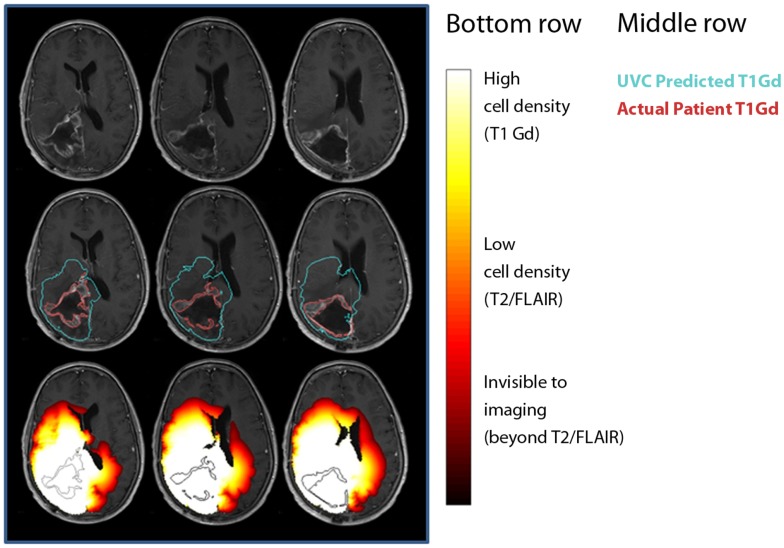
Comparisons between untreated virtual controls and post-treatment MRI scans. First row: post-treatment MRI. Second row: contours showing measured tumor on T1-Gd-enhanced scan (red) and UVC prediction of T1-Gd area (aqua). Third row: UVC tumor cell densities overlaid (white, high cell density; red, low cell density) on scan with T1-Gd measured tumor outline (black).
